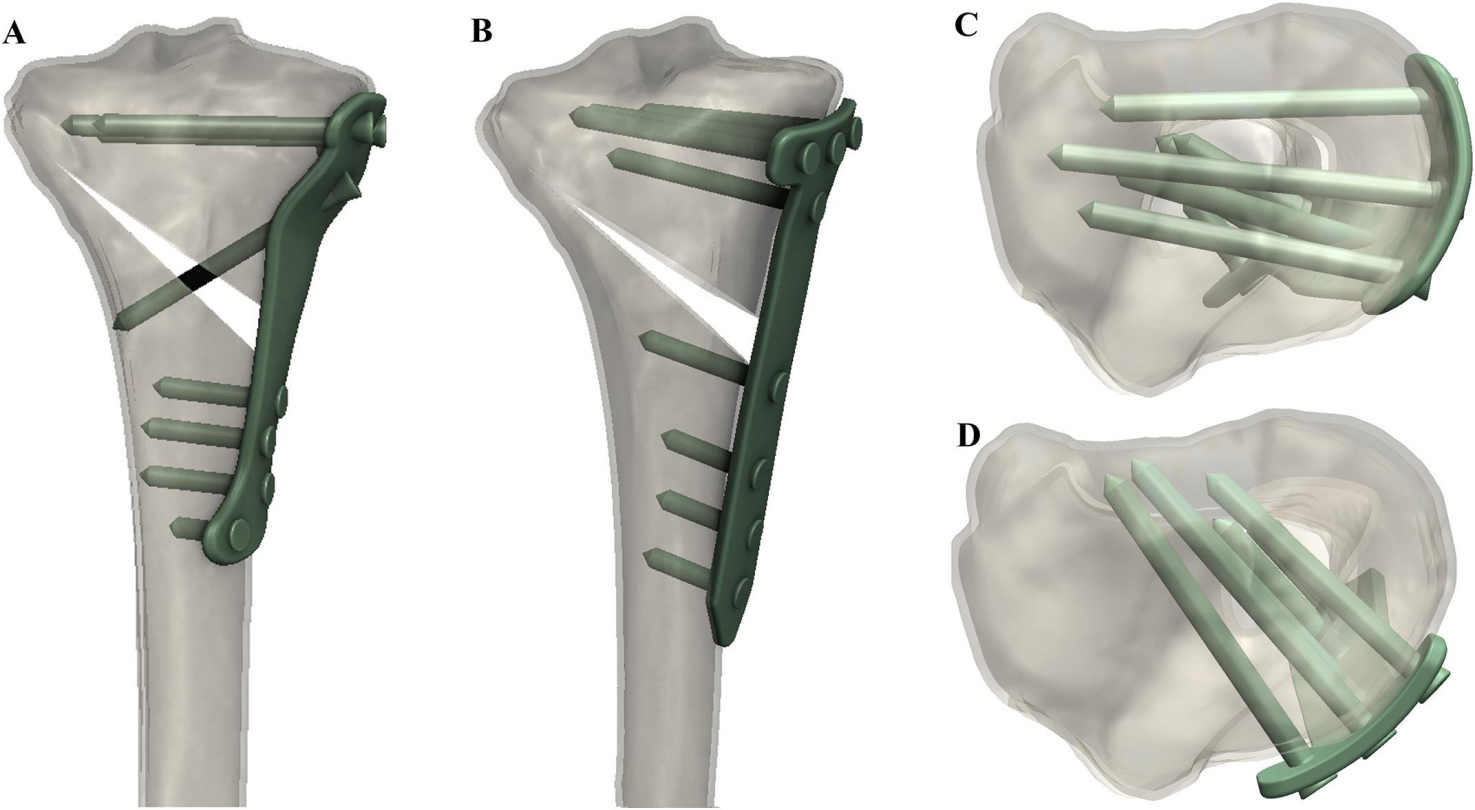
3D-reconstructed CT images showing (A) coronal view with the novel locking plate, (B) coronal view with the T-shaped locking plate, (C) axial view with the novel locking plate, and (D) axial view with the T-shaped locking plate.
